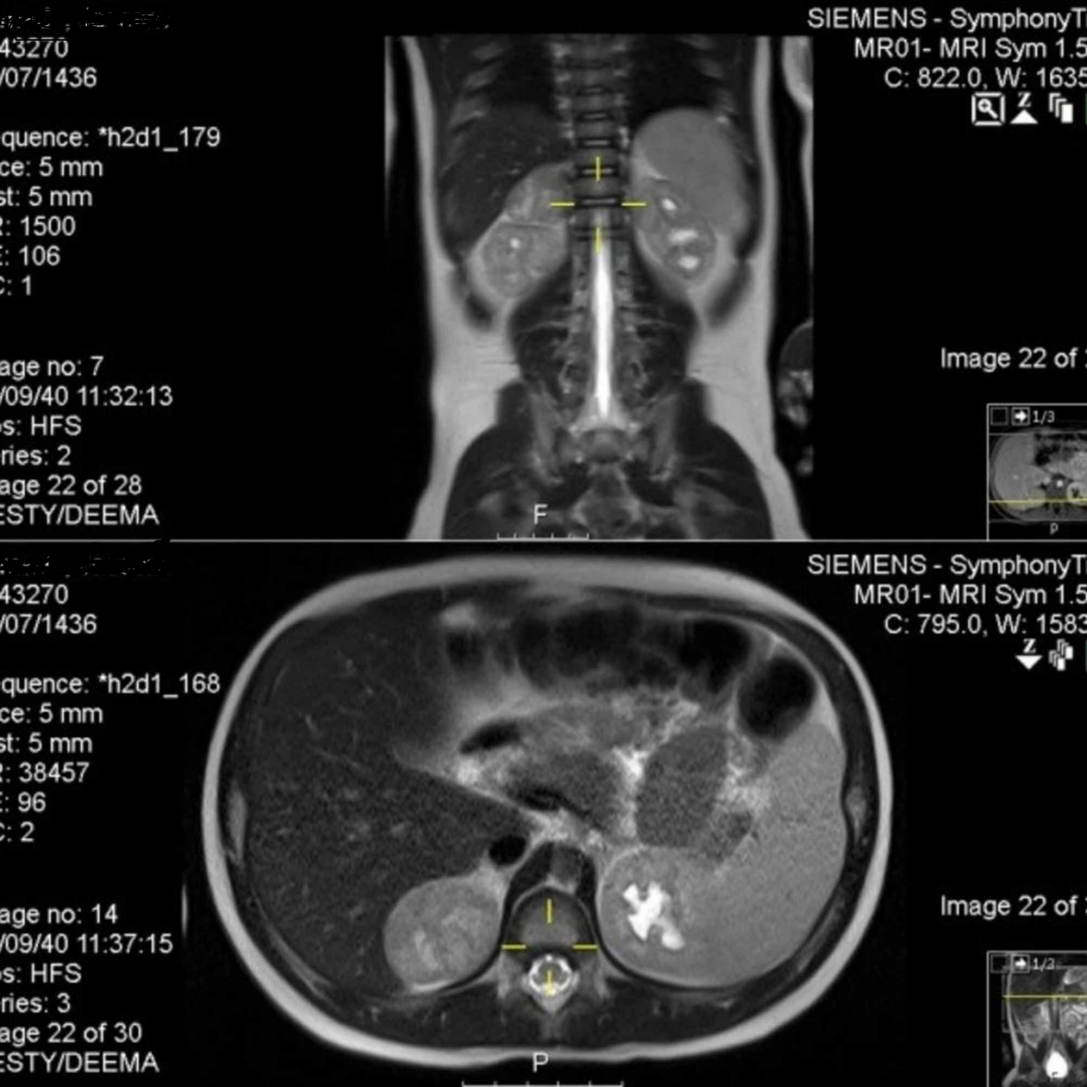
Magnetic resonance imaging showing an adrenal tumor
